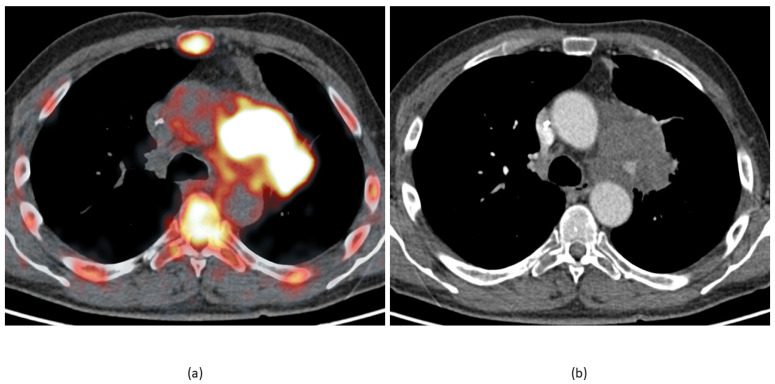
The same patient and study as in Figure 1 and Figure 2. (a) Fusion image shows increased uptake in the aortic arch walls and a lung mass infiltrating the mediastinum and forming a close contact with the vessel wall. This makes a direct measurement of the vessel wall’s metabolic activity prone to unintended errors (i.e., part of the tumor tissue may get into a vessel region of interest). (b) The arterial phase of the contrast-enhanced CT at the same level shows almost no changes in the aorta walls.
